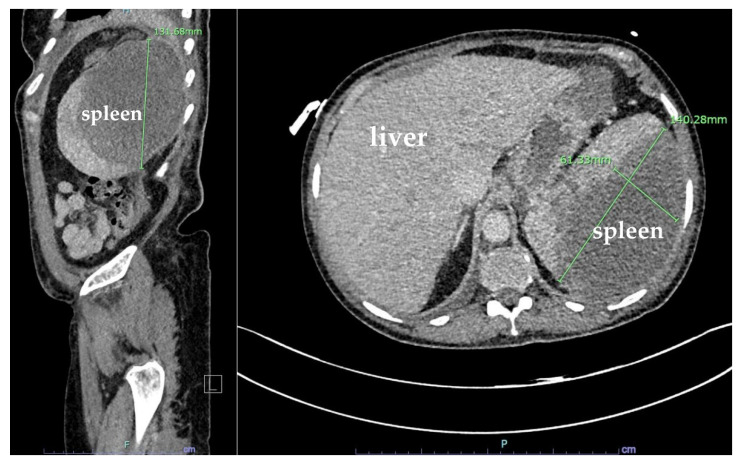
Coronal and sagittal plane computed tomography angiogram (CTA) scans with IV contrast of the abdomen and pelvis showing large splenic infarction with an increase in the size of splenic subcapsular hematoma, measuring up to approximately 14.0 × 13.2 × 6.1 cm, with a mass effect on the splenic parenchyma and an increase in the moderate-volume hemoperitoneum.
